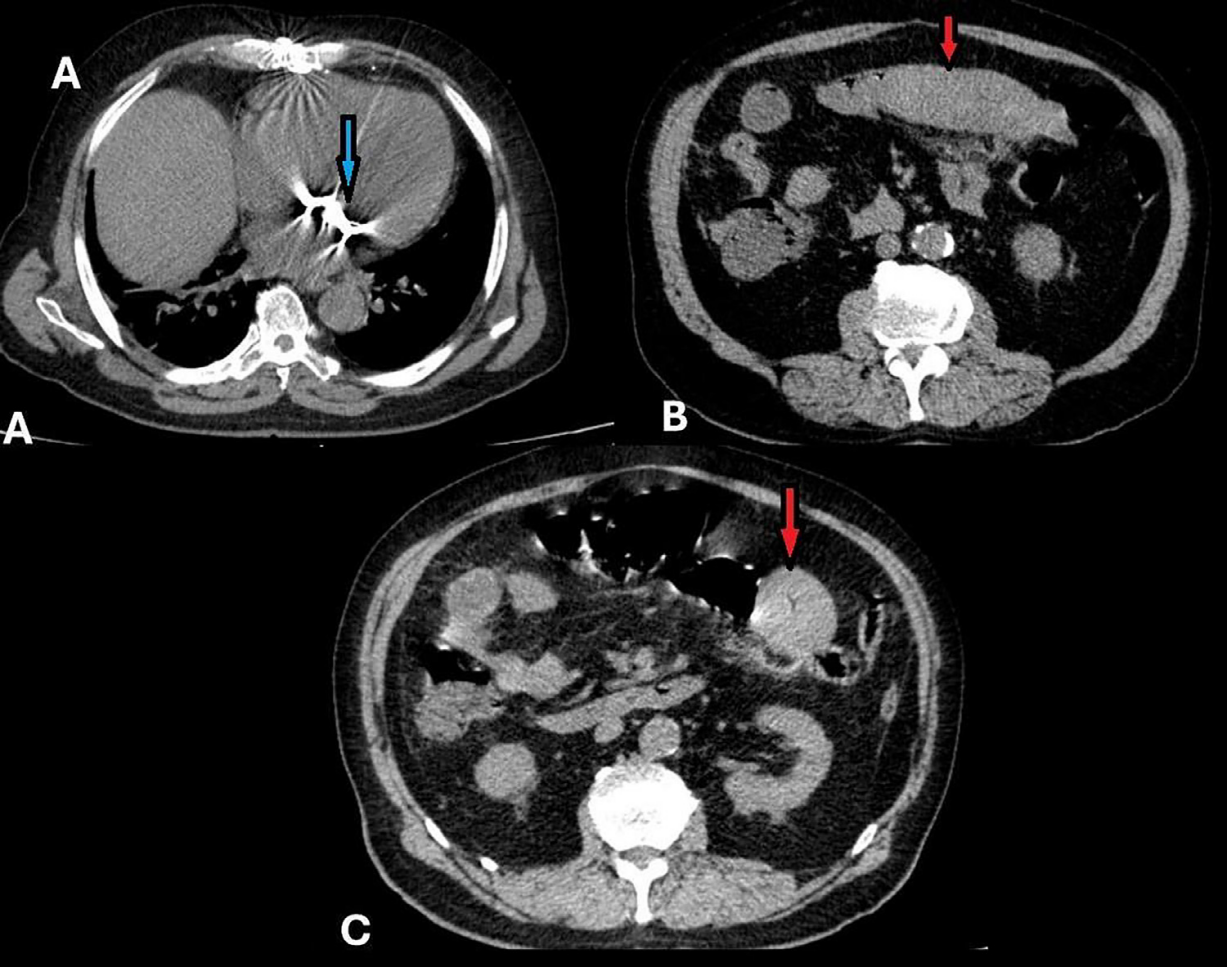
Nonenhanced Abdominopelvic computed tomography (CT) images. (A) Evidence of prior sternotomy and mitral valve replacement is visible (Blue arrow). (B and C) The jejunal loop shows hyperdense long-segment circumferential wall thickening, with adjacent mesenteric haziness and fluid (red arrows).
